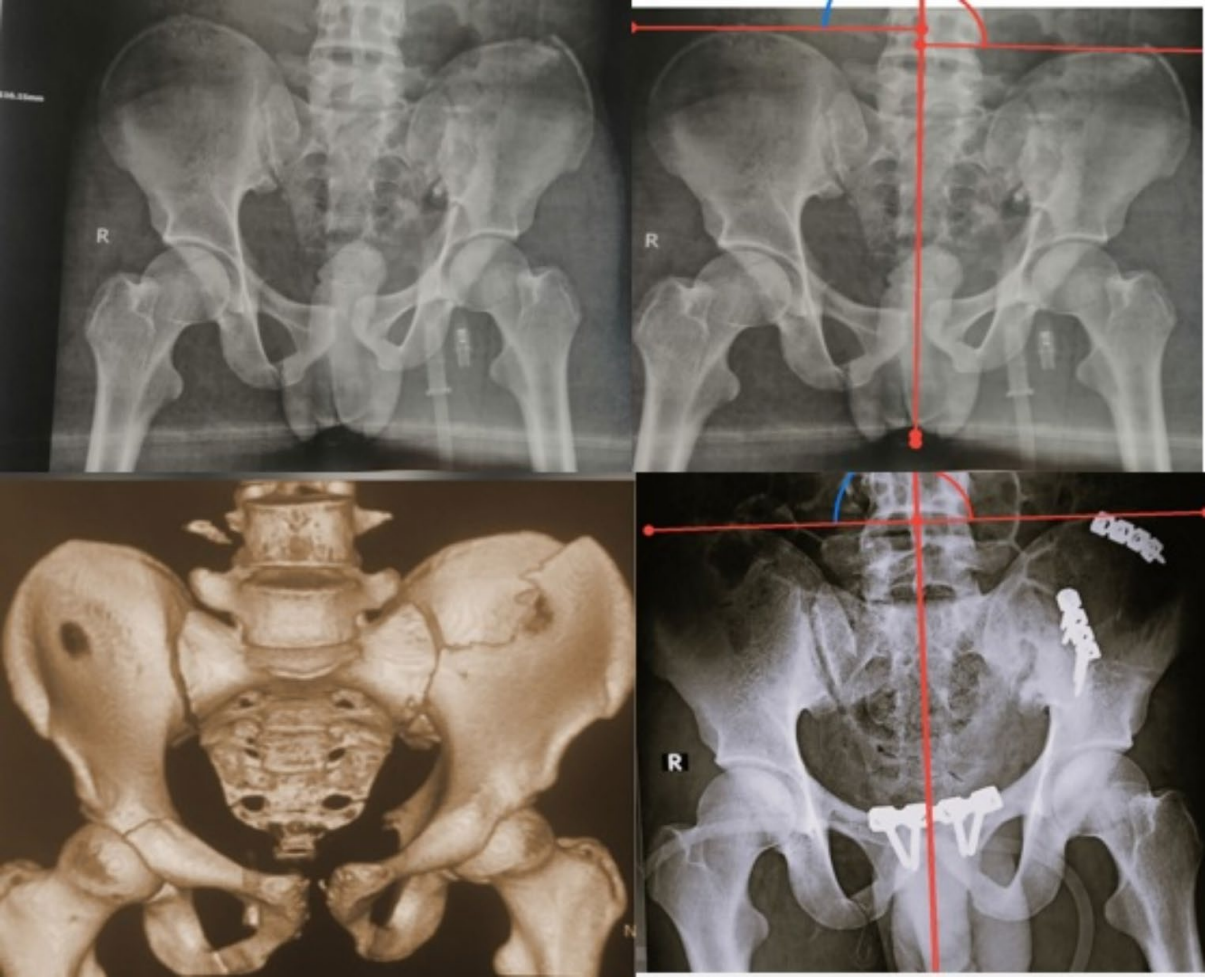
Preoperative and postoperative plain radiograph showing symphyseal plate fixation with measurement of vertical pelvic displacement using Henderson horizontal reference lines
